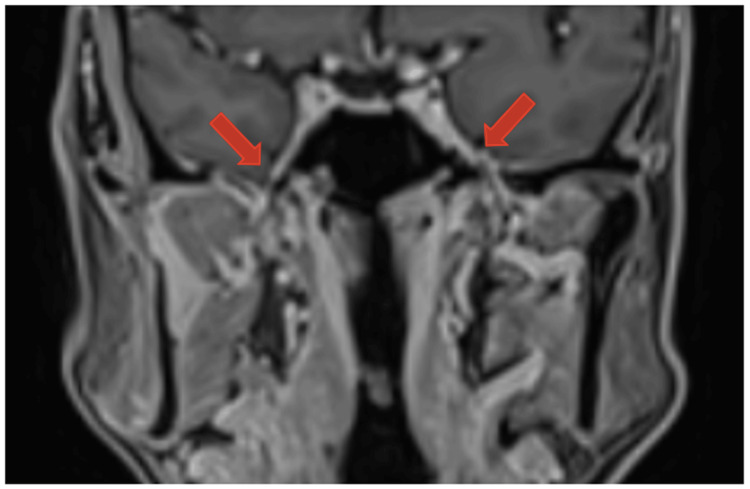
MRI neck coronal T1 fat-saturated (T1FS) post gadolinium image. Bilateral symmetrical appearance of the mandibular division of trigeminal nerves, indicating no intracranial extension of the perineural spread.
